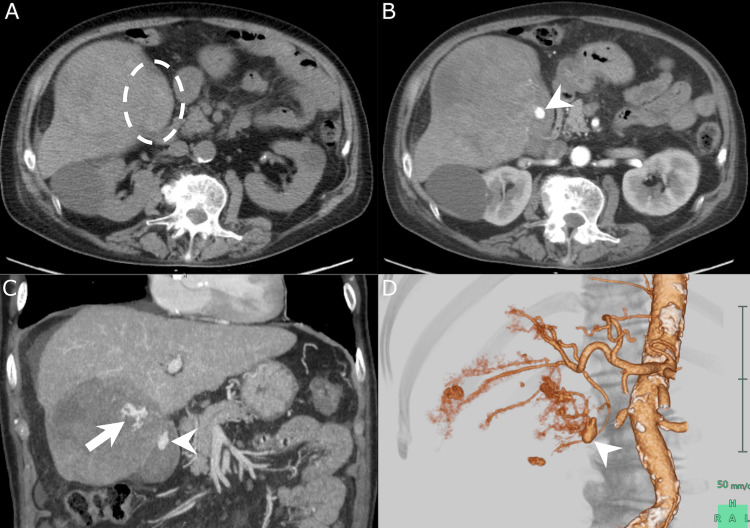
Non-contrast and contrast-enhanced CT image (A) A non-contrast-enhanced CT image demonstrates a hematoma in the gallbladder (white dotted circle). (B) An early phase contrast-enhanced CT image demonstrates a pseudoaneurysm in the gallbladder (white arrowhead). (C) A coronal maximum intensity projection image demonstrates extravasation of contrast media within a large hepatic tumor (white arrow) and a pseudoaneurysm in the gallbladder (white arrowhead). (D) A three-dimensional volume-rendered CT angiography image demonstrates a cystic artery pseudoaneurysm (white arrowhead). CT, computed tomography
